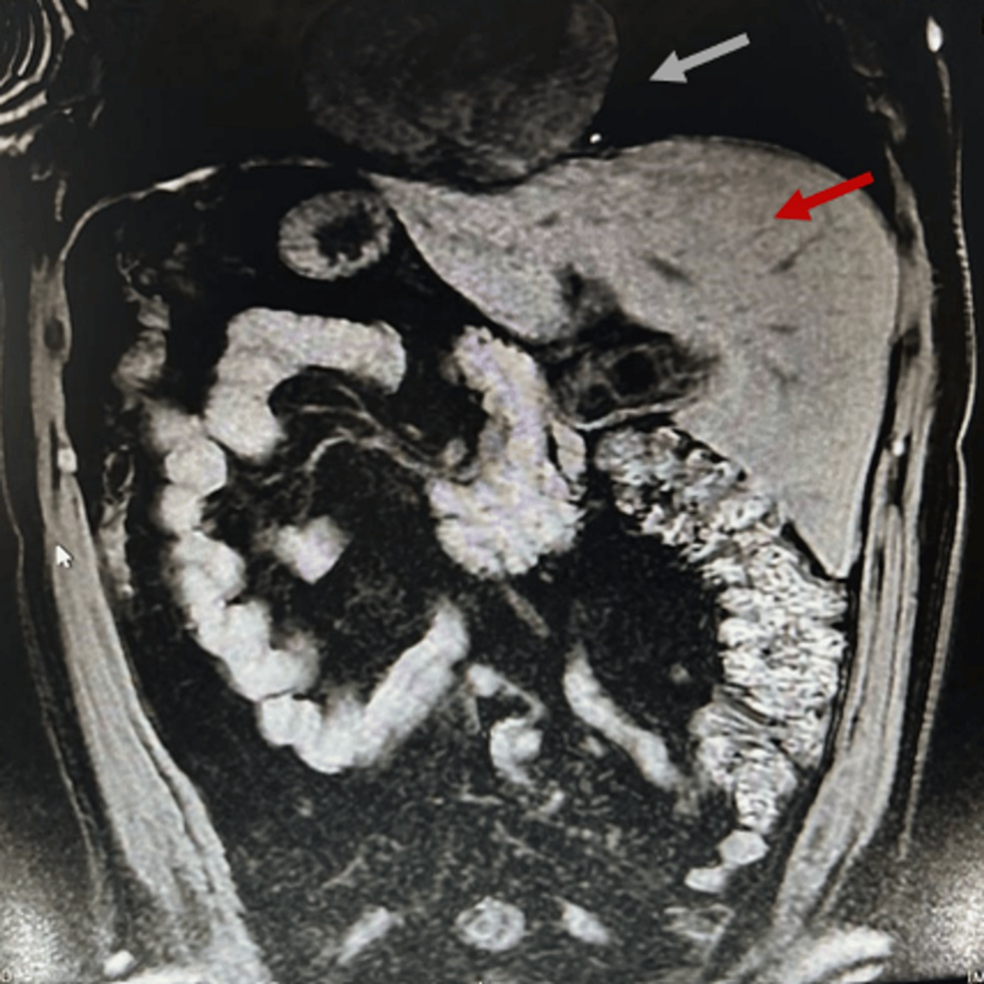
MRI, T1 weighted, coronal plane Grey arrow: Normal cardiac position Red arrow: Liver in the sinistroposition confirming *situs inversus*
